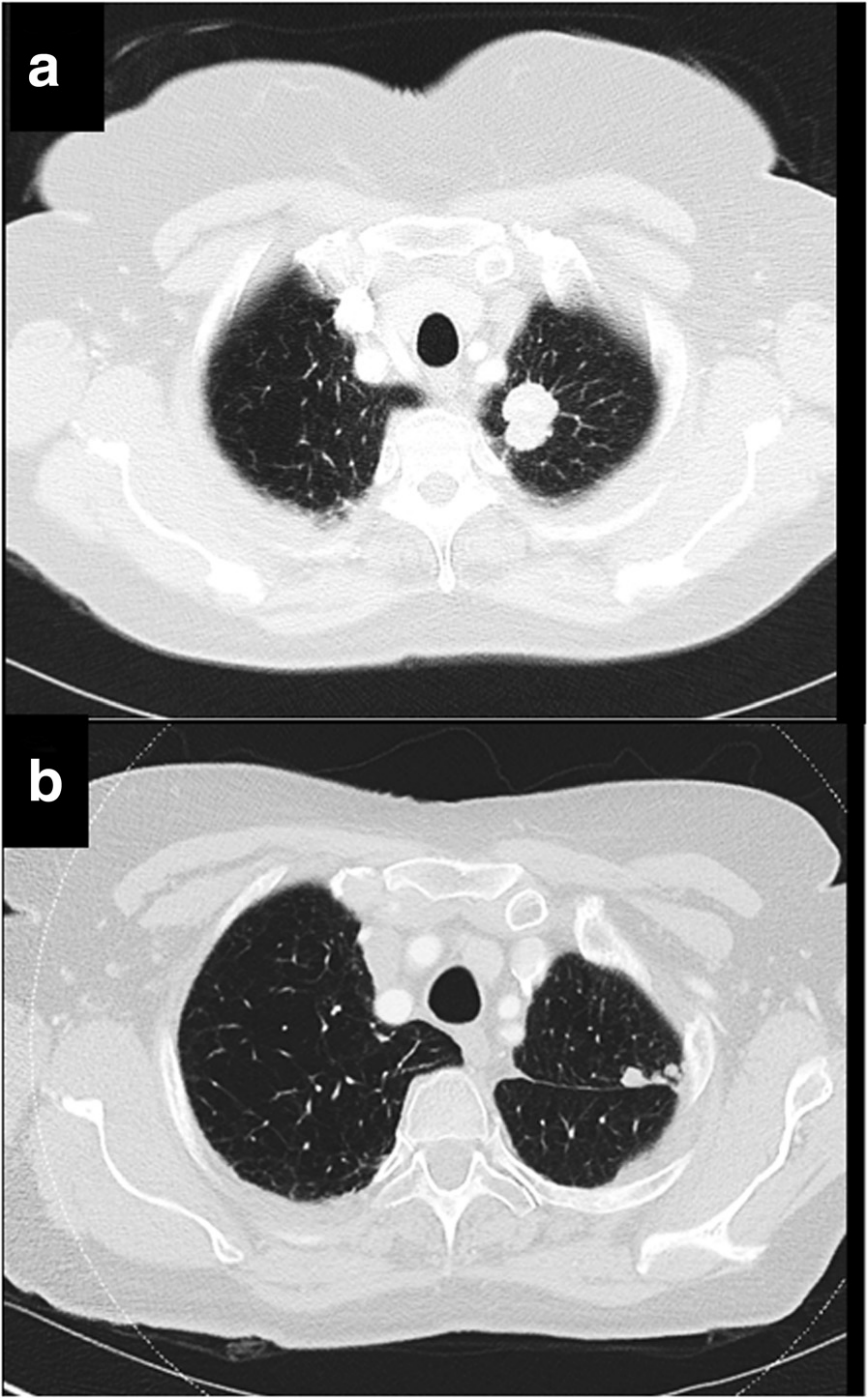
The potential for prognostic biomarkers to stratify care for patients with NSCLC. CT showing left upper lobe NSCLC at initial staging a. Based on current practice, the patient underwent surgery without adjuvant chemotherapy. CT performed 25 months later shows local recurrence b. A biomarker deployed at staging may have categorised the patient as high-risk for recurrence, implying a potential benefit from adjuvant chemotherapy
